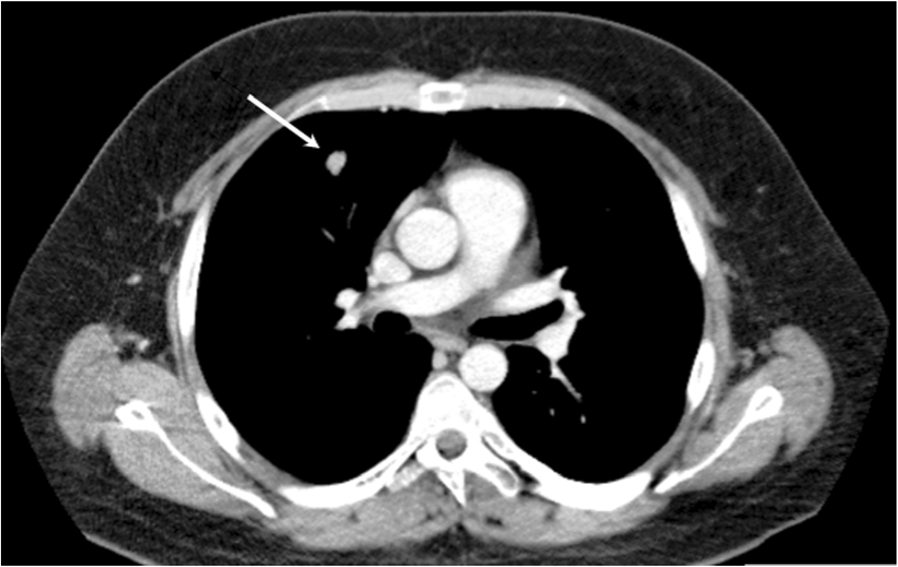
Investigation with computed tomography shows a 12×9×14mm tumor in the right lower pulmonary lobe in a 22-year-old man with aggressive adrenocorticotropic hormone-dependent Cushing’s syndrome. Tumor indicated by an arrow.
